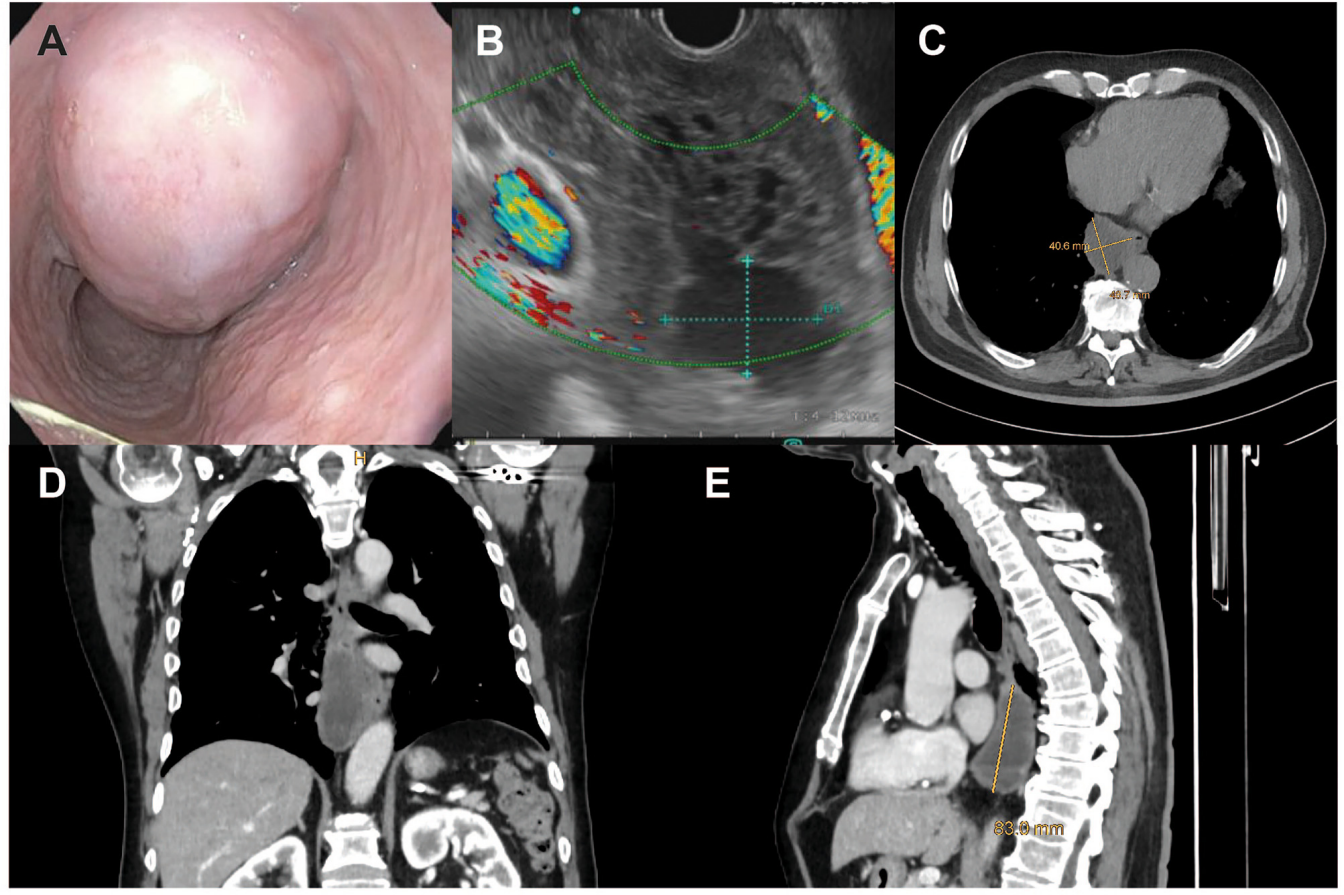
Case of a male patient with a large (9 × 5 cm) esophageal GIST. Endoscopic (A) and endosonographic (B) view, as well as transverse (C), coronal (D) and sagittal (E) computed tomography planes of the tumor.
